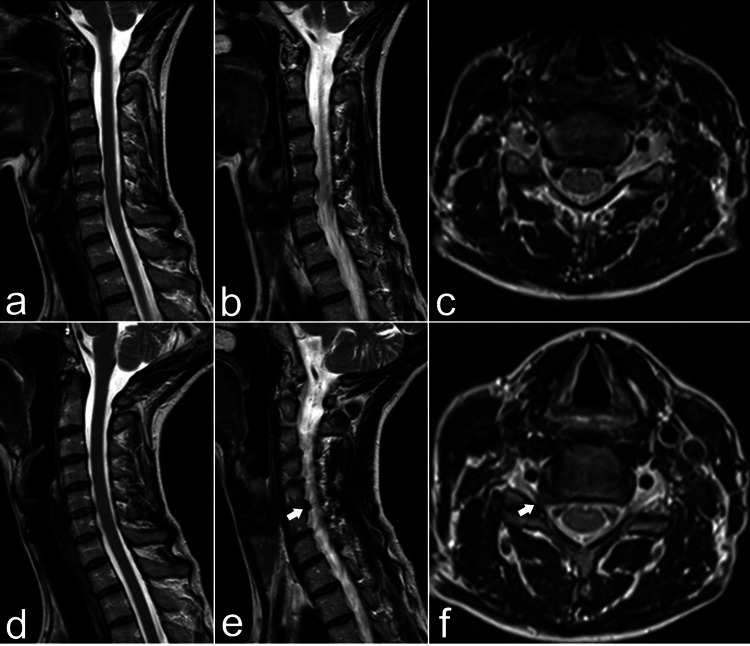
T2-weighted MRI in the neutral position: (a) sagittal, (b) right parasagittal, and (c) T1.5-weighted axial images, with corresponding extension images shown in (d-f). In the neutral position, there is no significant spinal canal or foraminal stenosis. However, with neck extension, a prominent disc protrusion is observed, resulting in severe right 6 foraminal stenosis (arrow).
